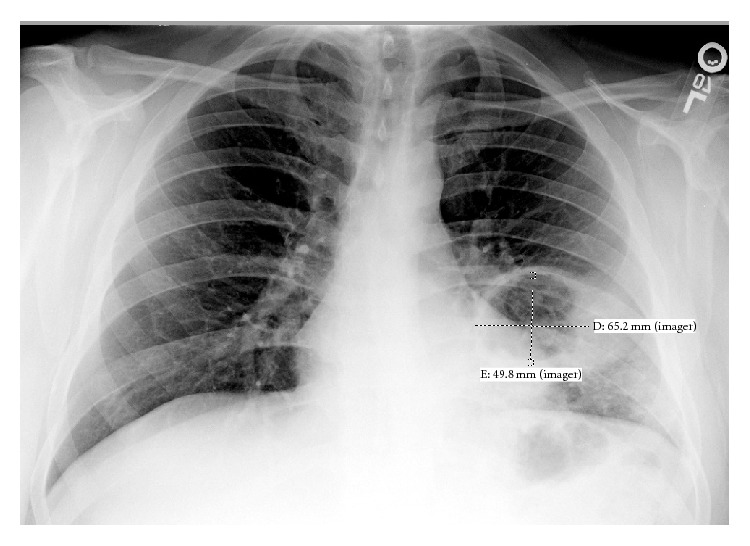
Chest X-ray on admission with 6.52 cm by 4.98 cm cavitary lesion.
